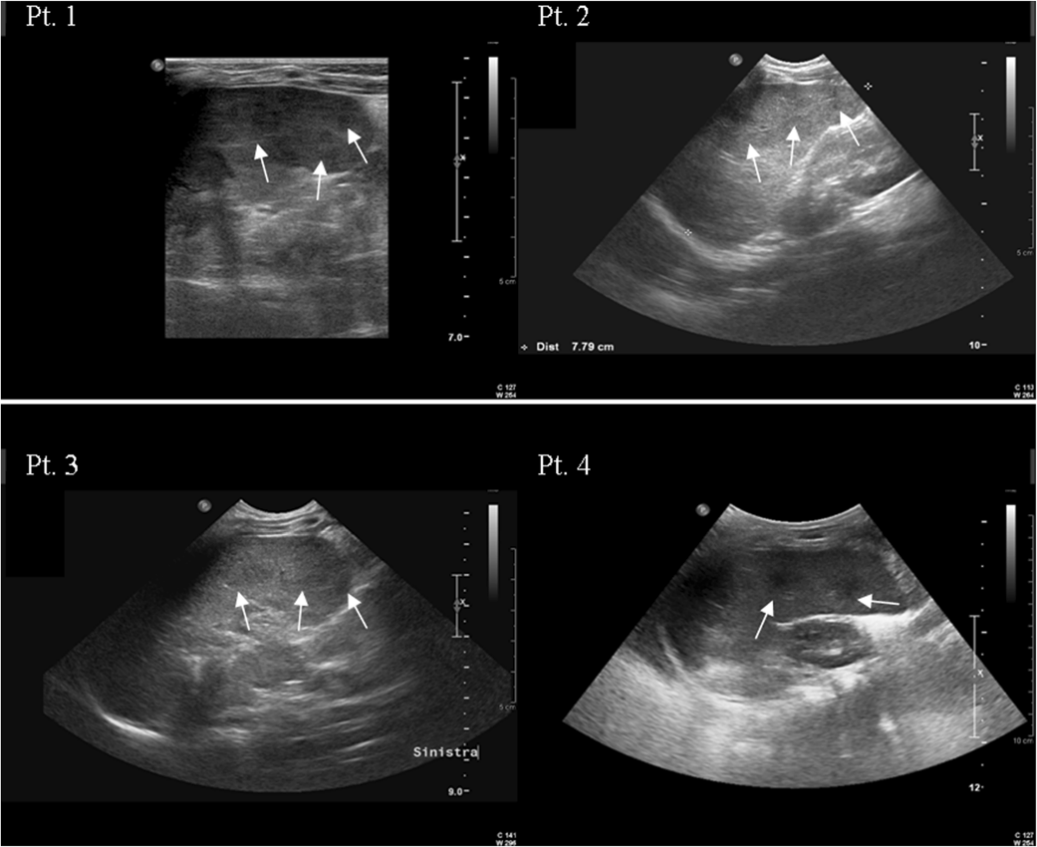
Spleen sonography of 4 patients showing splenomegaly with multiple small iso-hypoechoic nodules (arrows).
